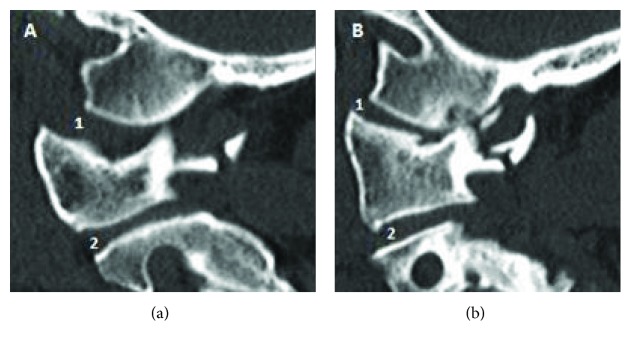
Cervical spine CT scan. (a) Widening and slight anterior subluxation of the right atlantooccipital joint (1) with preserved anatomy of C1–C2 lateral mass joint (2). (b) Slight anterior subluxation of the left atlantooccipital joint (1) and widening of C1–C2 lateral mass joint (2).
